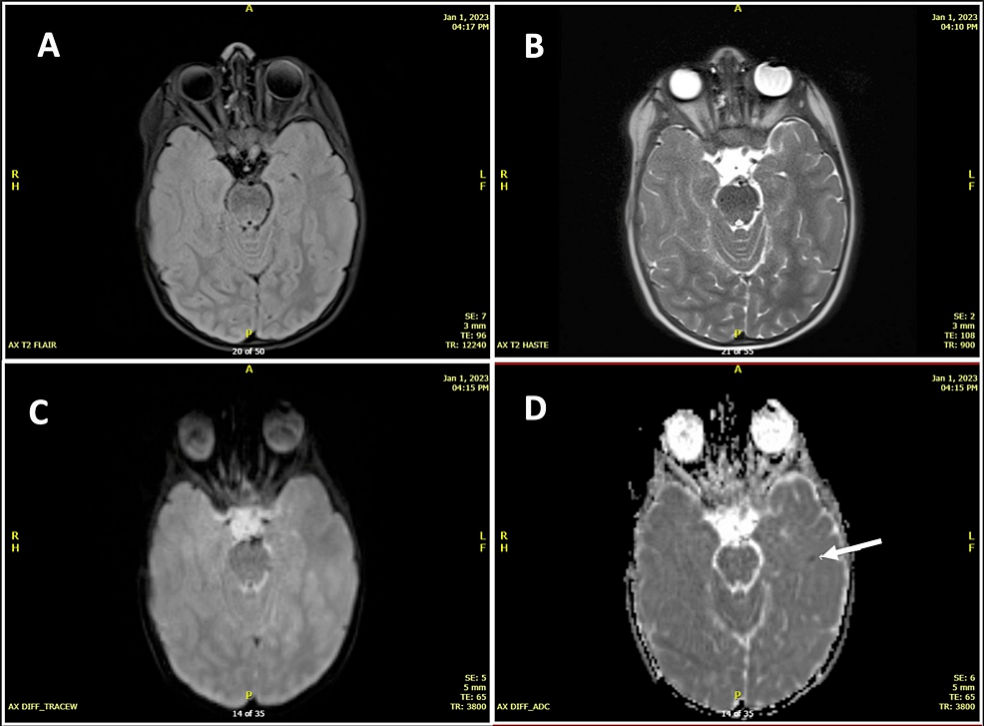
MRI brain without contrast. A. Axial T2 fluid-attenuated inversion recovery (FLAIR), B. Axial T2 half-Fourier acquisition single-shot turbo spin echo (HASTE), C. Axial trace diffusion (TRACEW), D. Axial apparent diffusion coefficient (ADC), Arrow denoting the punctate area of restricted diffusion in the left temporal lobe.
